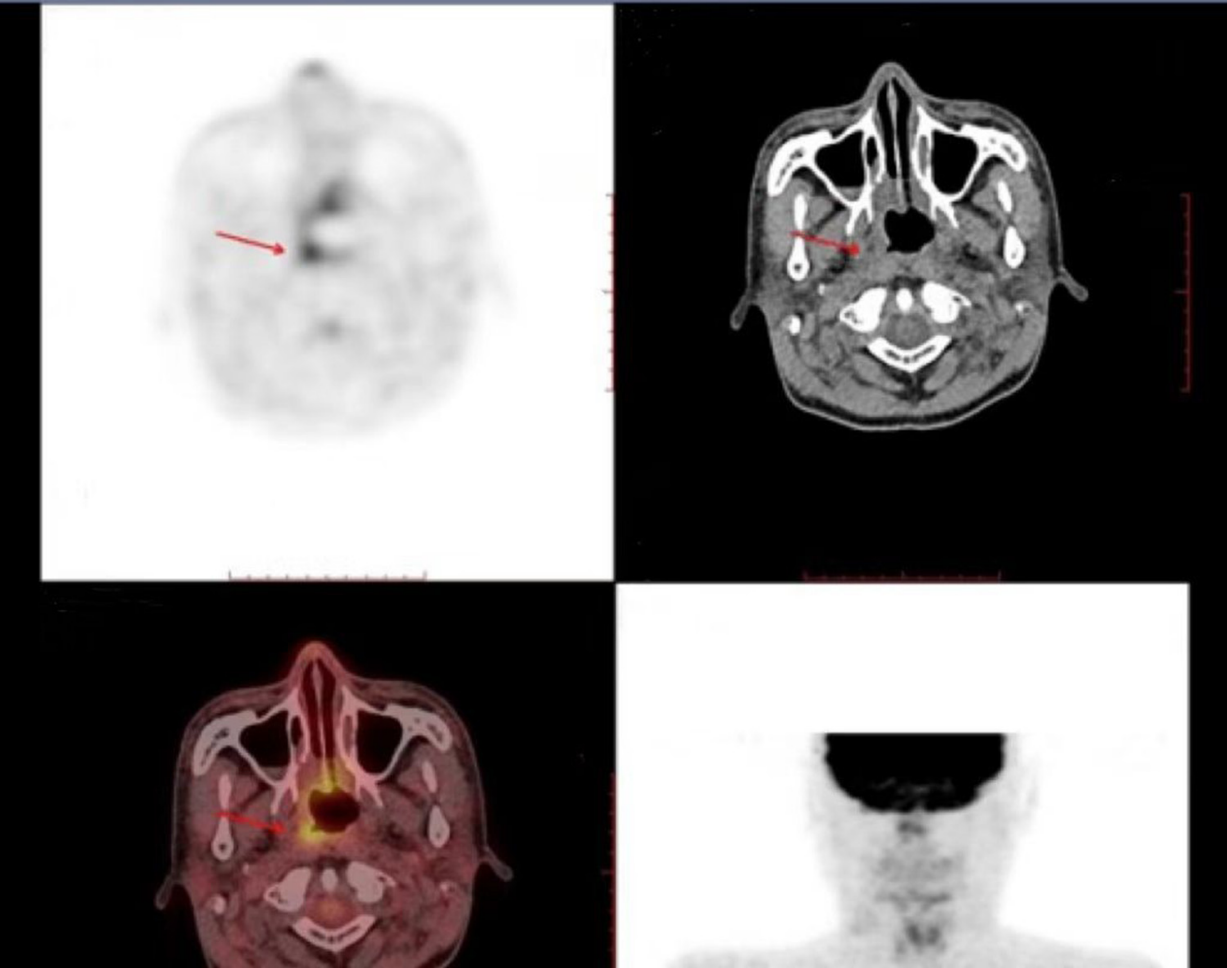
Positron emission tomography-computed tomography scans. The arrow shows that the mass significantly shrank.
